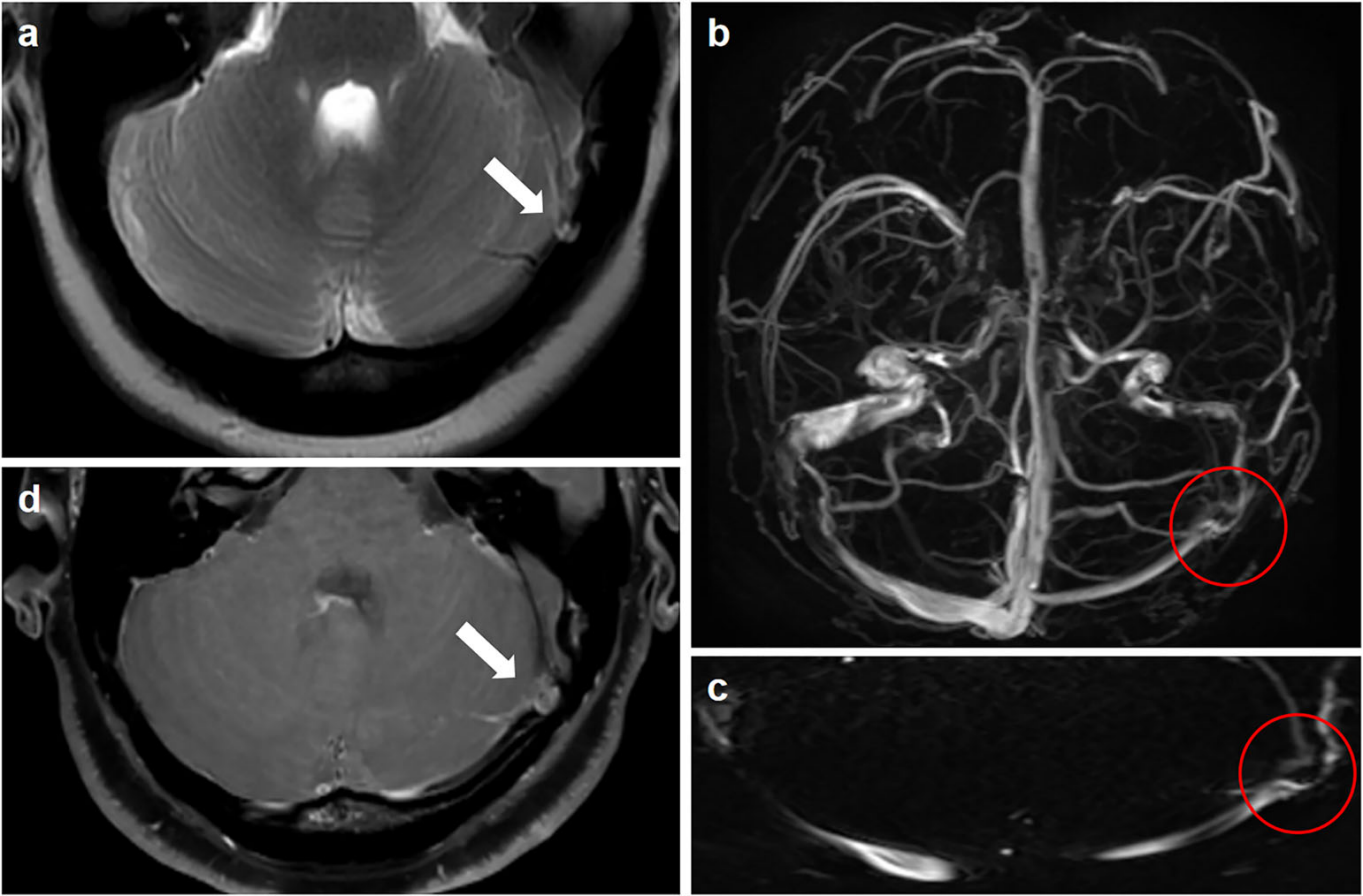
Classical signs suggestive of intrinsic stenosis. (a) Axial T2‐weighted magnetic resonance imaging (MRI) showing an arachnoid granulation (arrow). (b) Inferior reconstruction of magnetic resonance venography (MRV) demonstrating intrinsic transverse venous sinus stenosis from an arachnoid granulation (red circle). (c) Curved reformat of the dural venous sinus from the MRV showing that intrinsic stenosis may appear as discontinuous points of stenosis from an arachnoid granulation (red circle). (d) High‐resolution 3D T1‐weighted SPACE showing unilateral sinus stenosis caused by the protrusion of an arachnoid granulation into the lumen of the sinus (arrow).
